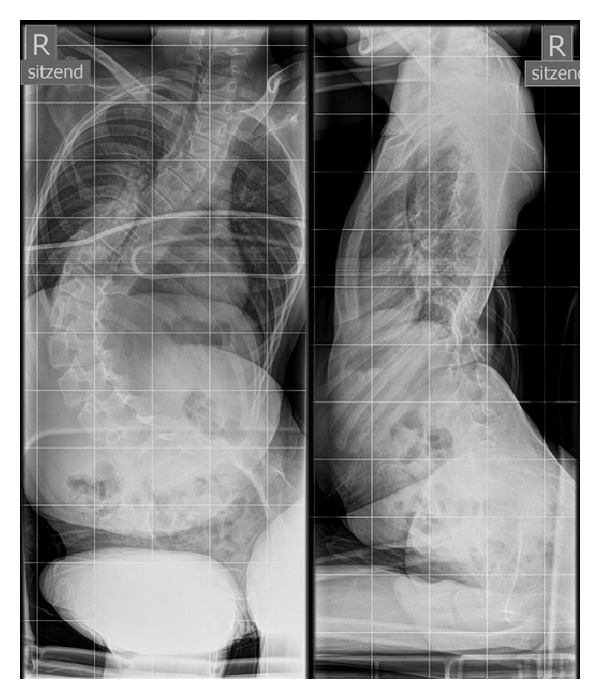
Male PD patient, 13 years old. Scoliosis right-convex C-shaped 90° Cobb Th6/L1/L5, before surgical spine stabilization. Pelvic obliquity 40° to the right. AP and sagittal radiograph.
